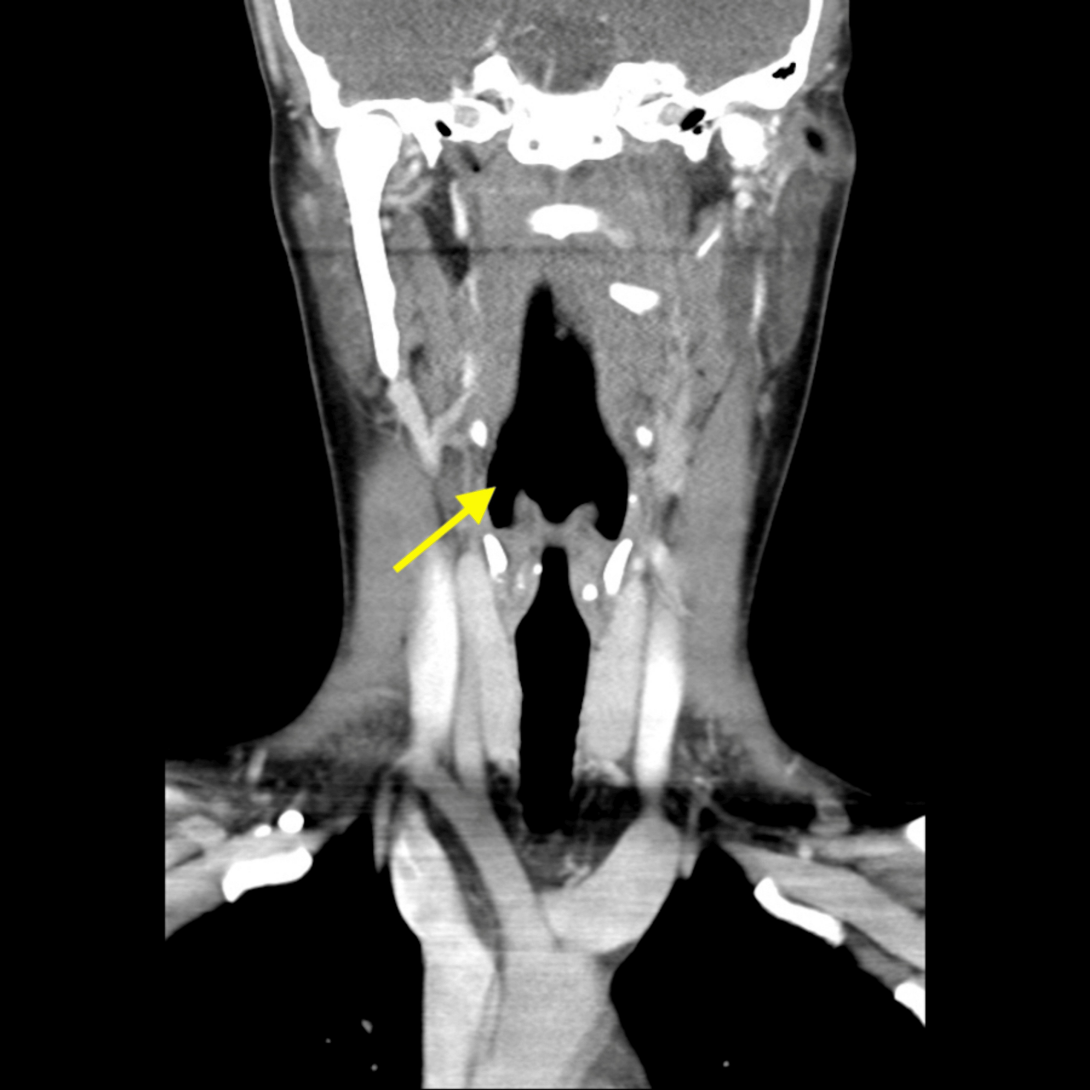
CT scan of neck, coronal view Showing a clear tracheal air column with normal epiglottis and no prevertebral soft tissue swelling. Arrow demonstrating a patent airway.
